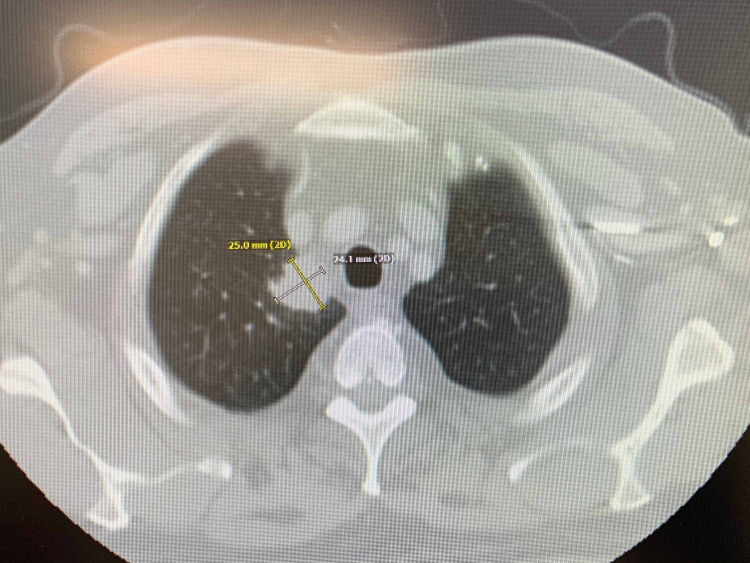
CT chest with contrast Irregular mass concerning for malignancy abutting the right mediastinum within this upper lobe measuring 2.3 x 3 cm. CT: computed tomography
